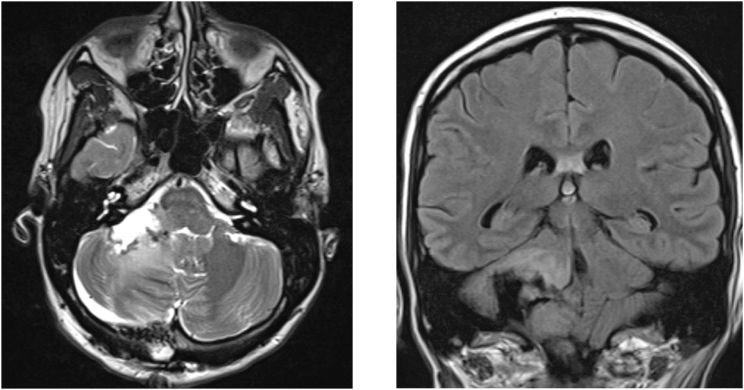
Postoperative MRI without contrast, T2 axial (left) and T2 TIRM coronal (right).
